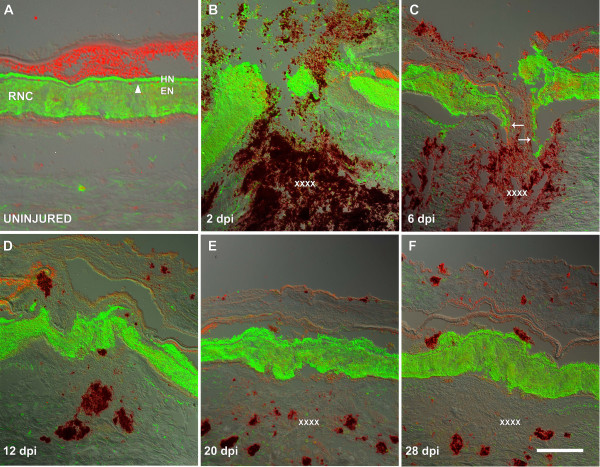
RN1 labeling of the regenerating radial nerve cord. Longitudinal tissue sections of (A) uninjured and regenerating radial nerve cords at (B) 2, (C) 6, (D) 12, (E) 20 and (F) 28 days post injury (dpi) were labeled with the monoclonal antibody RN1. (A) Labeling in the uninjured RNC is highly specific to the nervous components, both ectoneural (EN) and hyponeural (HN) component while the connective tissue band remains unlabeled (arrowhead). (B) At 2 dpi nerve fiber debris is visible around the injury site and the nerve stumps can be seen to be highly disorganized. (C) By 6 dpi, the RNC stumps have organized into club-shaped structures from which some nerve fibers can be observed to be extending (arrows). (D) By 12 dpi the injury gap has been filled with nervous tissue that now forms a continuous extension that joins the stumps. (E) By 20 dpi the RNC still appears slightly disorganized and the CT band is not present along the entire length of the regenerated RNC. (F) At 28 dpi, the RNC has recovered much of the structure and organization found within non-injured RNC including the connective tissue that separates the EN and HN components. EN-epineural component, HN-hyponeural component, RNC-radial nerve cord. X's denote the injury site; Bar = 300 μm.
